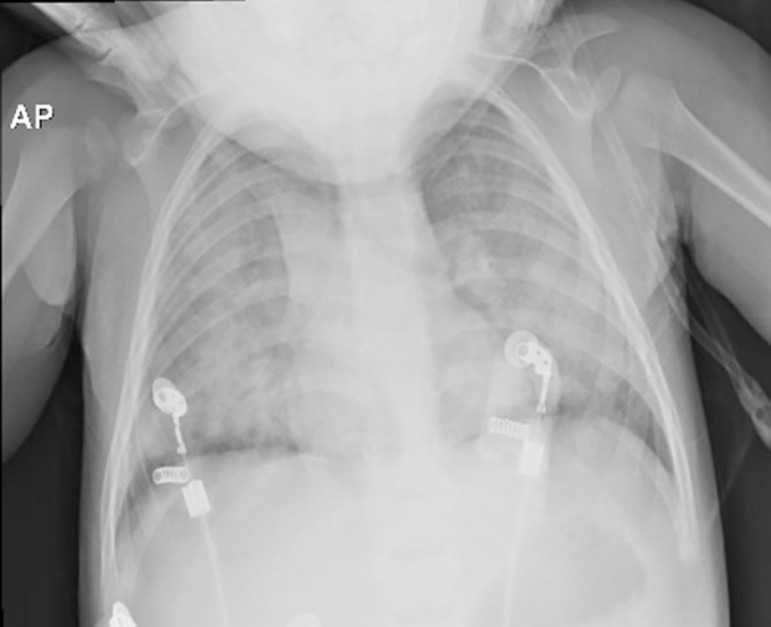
Chest X-ray at hospitalization (a few hours after the injury), in which
